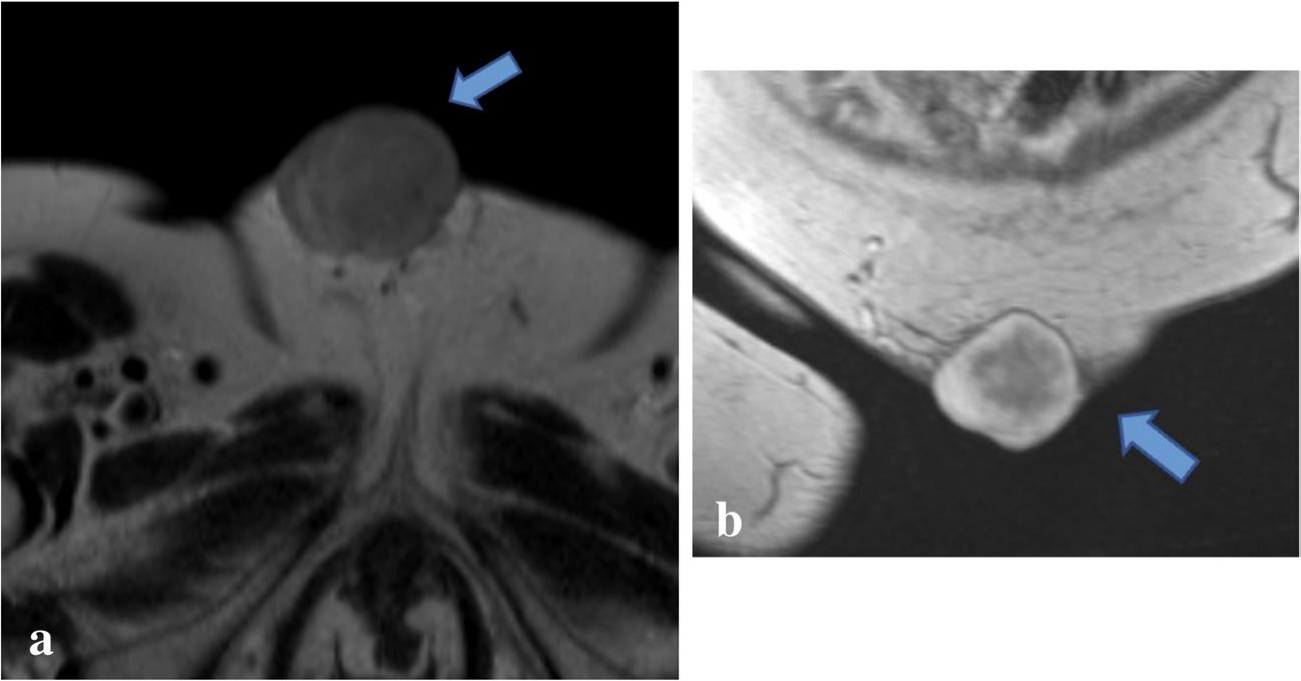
Seventy-eight-year-old female with dermatofibrosarcoma protuberans of the mons pubis. Axial T2 (a) and postcontrast coronal T1 (b) MRI images demonstrate an enhancing lobulated T2-intermediate signal mass centered at the skin of the mons pubis, measuring 4.5 × 4.1 cm. This tumor was resected and pathology-proven to be dermatofibrosarcoma protuberans, a rare sarcoma
